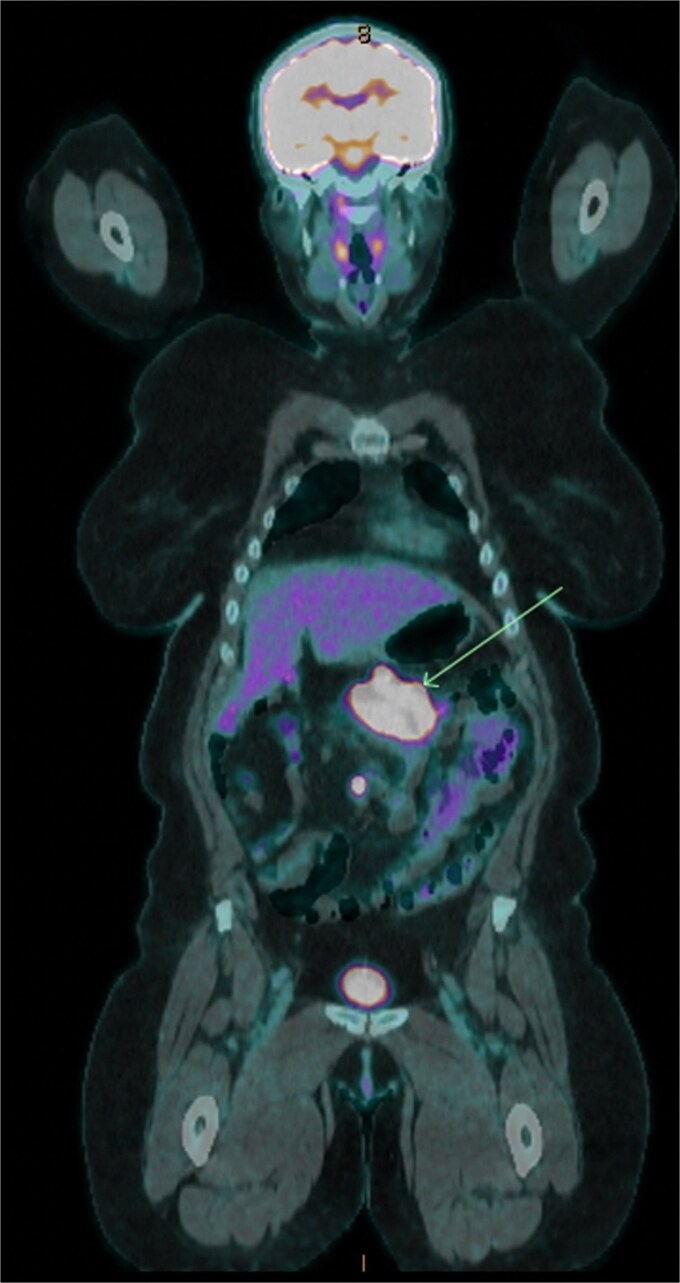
18F-FDG positron emission tomography/computed tomography showing transverse colonic recurrence (arrow).
